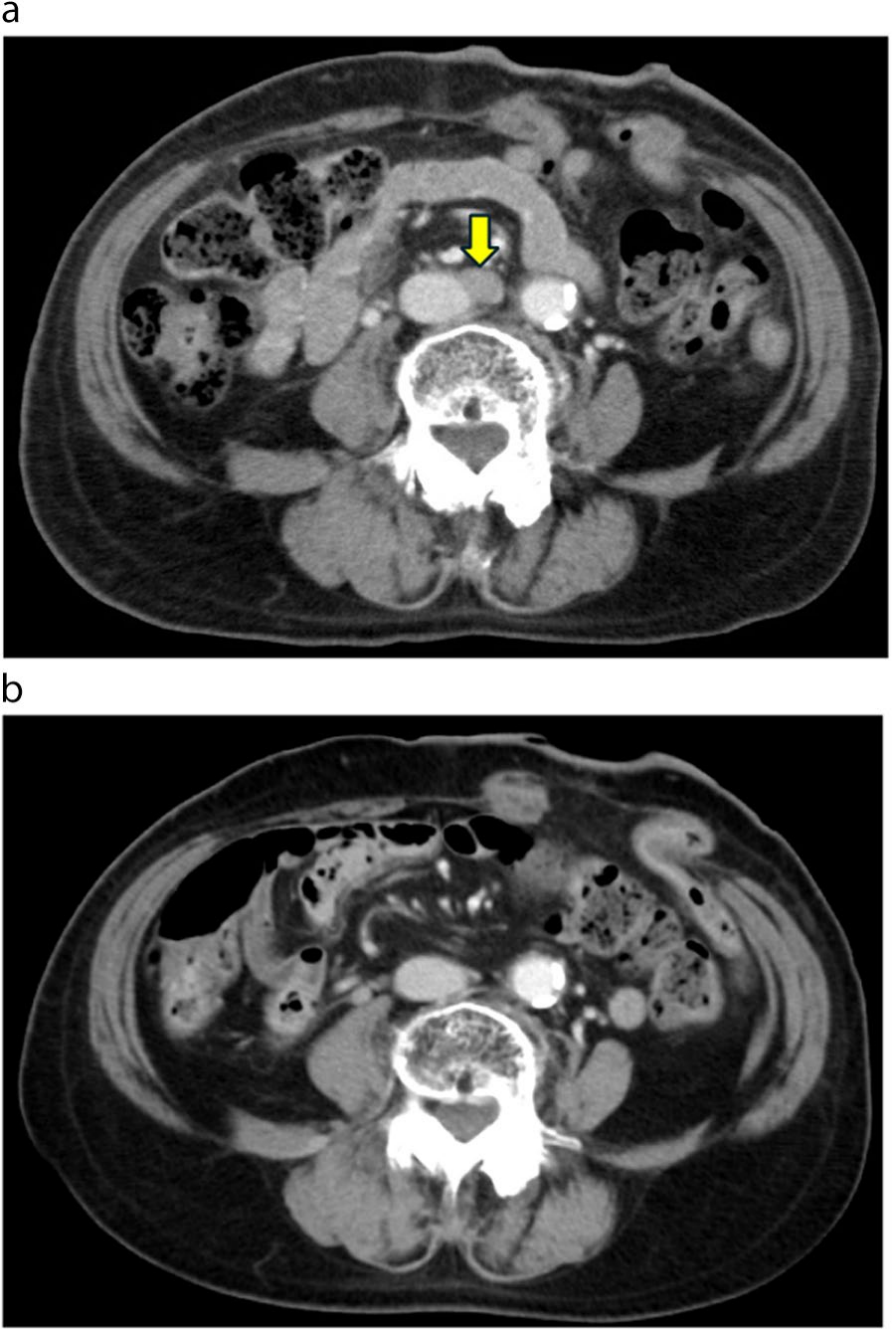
Pre- or post-DCF chemotherapy of the para-aortic lymph node status in an axial CT slice. a Para-aortic lymph node recurrence is observed (arrow). b After initiating DCF chemotherapy, para-aortic lymph node recurrence demonstrated CR. *DCF* docetaxel, cisplatin, and 5-fluorouracil
